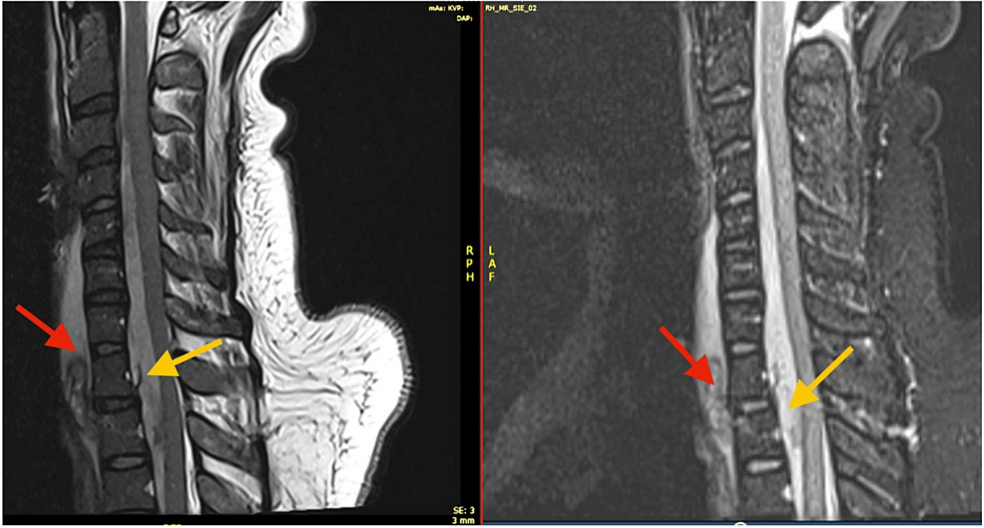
MRI SAG T2 and STIR images reveal large prevertebral (red arrow) and intraspinal epidural (yellow arrow) hematoma (C5-T2) with spinal cord compression. STIR: Short Tau Inversion Recovery; SAG: Sagittal
